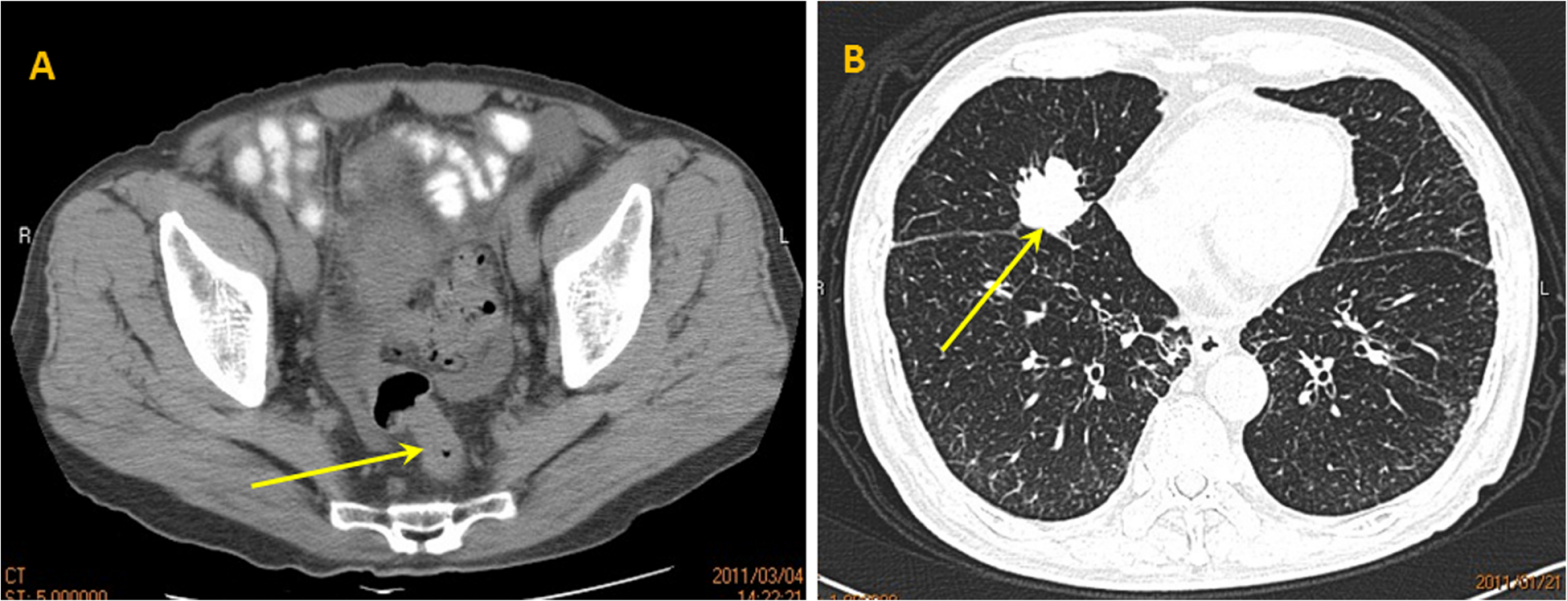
Computed tomography (CT) of the abdomen and chest. (A) Abdominal CT revealed wall thickening of the sigmoid colon, which is compatible with colon cancer (arrow). (B) Chest CT revealed a 3.2-cm nodule in the right middle lung field (arrow).
